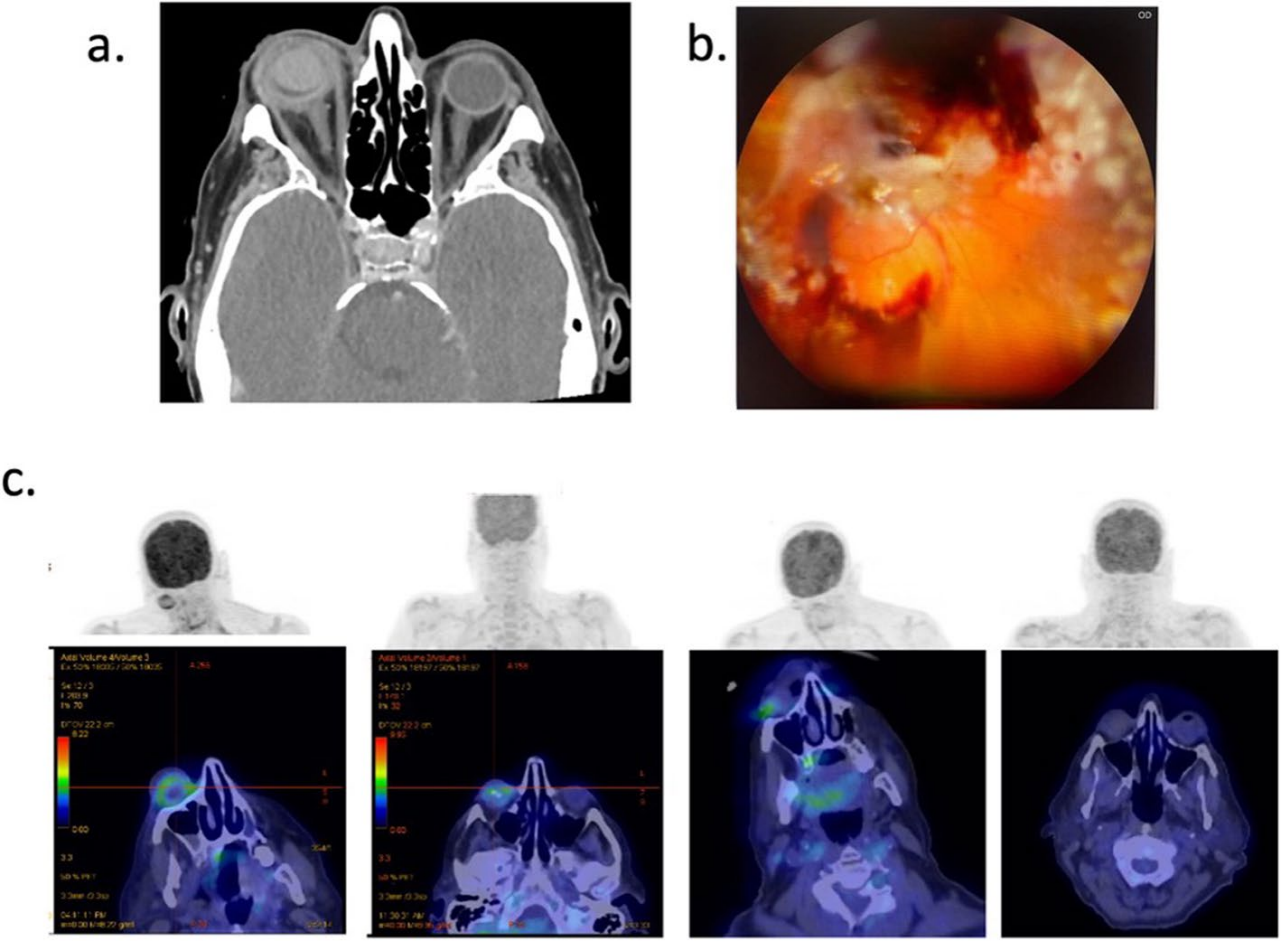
a Coronal computerised tomography scan of the orbit demonstrating circumferential thickening and enhancement around the entire right globe, vitreous haemorrhage, proptosis and retro-orbital intraconal inflammatory stranding. Normal CT appearance of the left globe. B Intraoperative right fundus photo of the right eye demonstrating large temporal retinal detachment, vitreous haemorrhage, superior area of retinitis and subretinal abscess extending inside superior arcade into macula. C Serial Fludeoxyglucose (FDG) positron emission tomography (PET) scans demonstrating FDG uptake and inflammatory changes in the right globe and subsequent interval improvement in right globe avidity. Images were obtained at approximately 1 month intervals from initial presentation
